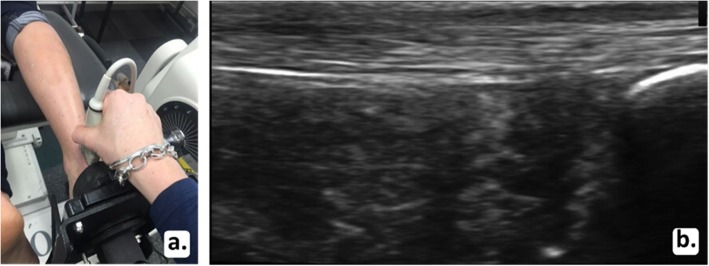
Second probe positioning (a) and resulting ultrasound image of calcaneal insertion of the Achilles tendon as indicated by the arrow (b)
